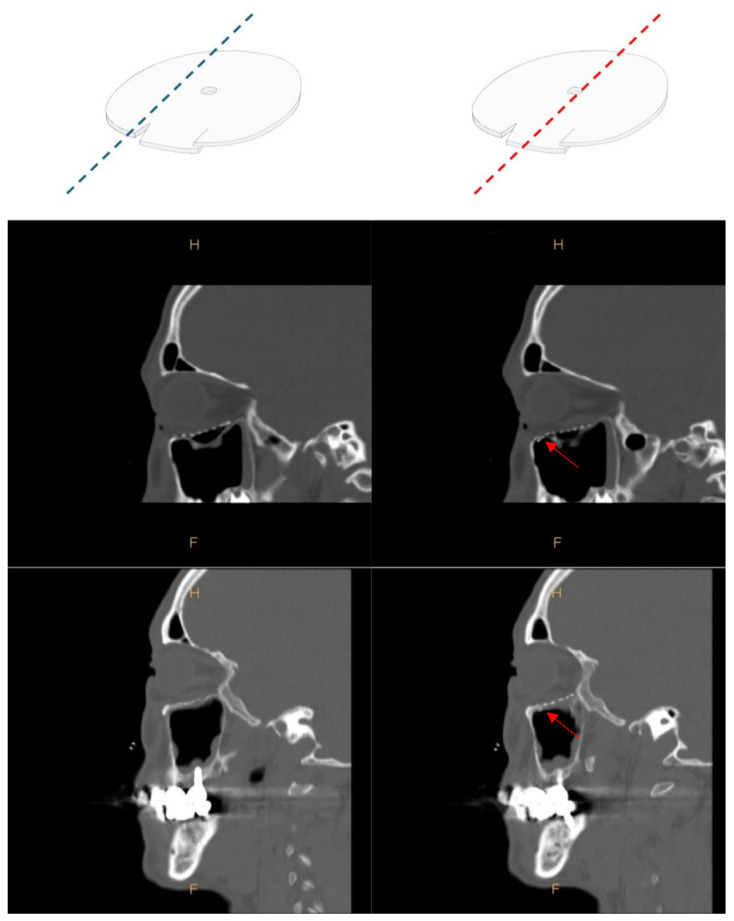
Tongue-in-groove implants as shown on CT. For intuitive understanding, we have attached the CT findings of the implant containing the titanium mesh. The pictures on the (left) are the CT results corresponding to the blue dotted line. It is confirmed that the implant is located above the intact bone. The pictures on the (right) are the CT results corresponding to the dotted red line. The groove in front of the implant (red arrow) is tucked into the underside of the intact bone.
